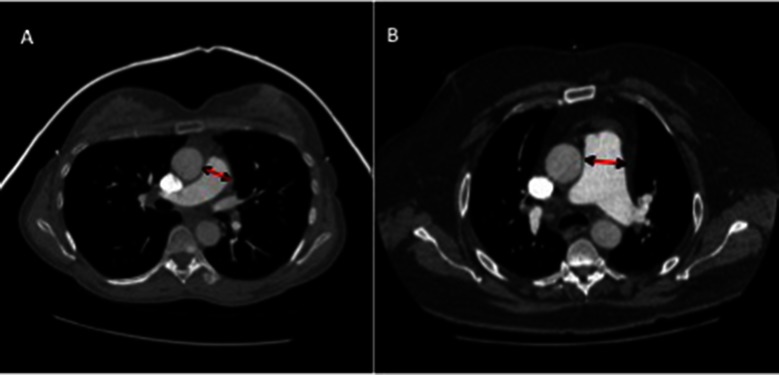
CT images of pulmonary artery diameter measurements in patients with (A) a patient without PH (mean pulmonary arterial pressure of 20 mmHg), (B) a patient with PH with moderate elevation in pulmonary arterial pressure (mPAP 54 mmHg). Diameter measured where largest and most consistent - proximal to bifurcation, perpendicular to direction of vessel.
